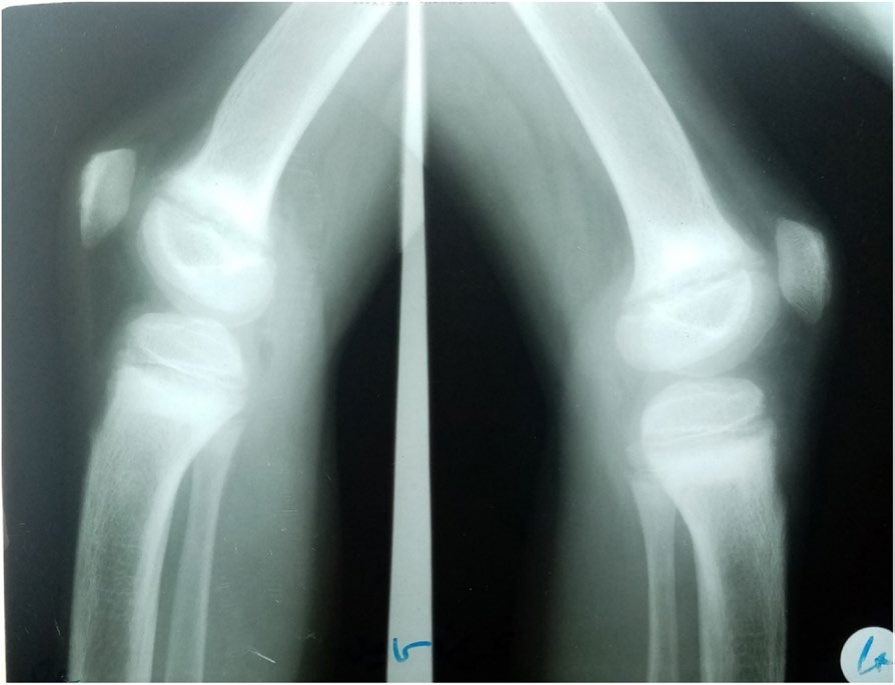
X-ray bilateral knees shows osteopenia, mild cupping, and fraying
